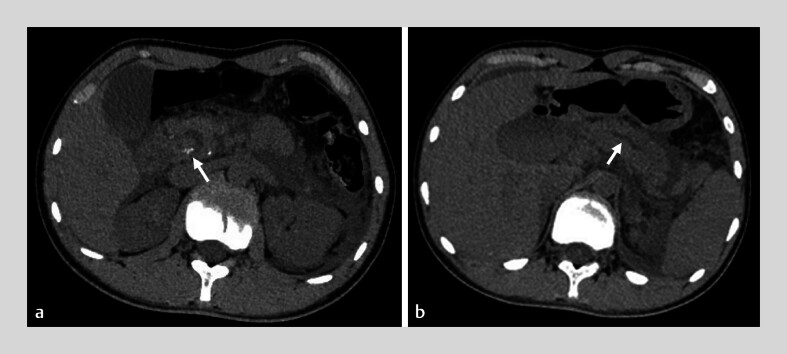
Computed tomography showed peripancreatic effusion, calcification, and dilation of the Wirsung and Santorini ducts (arrows).
